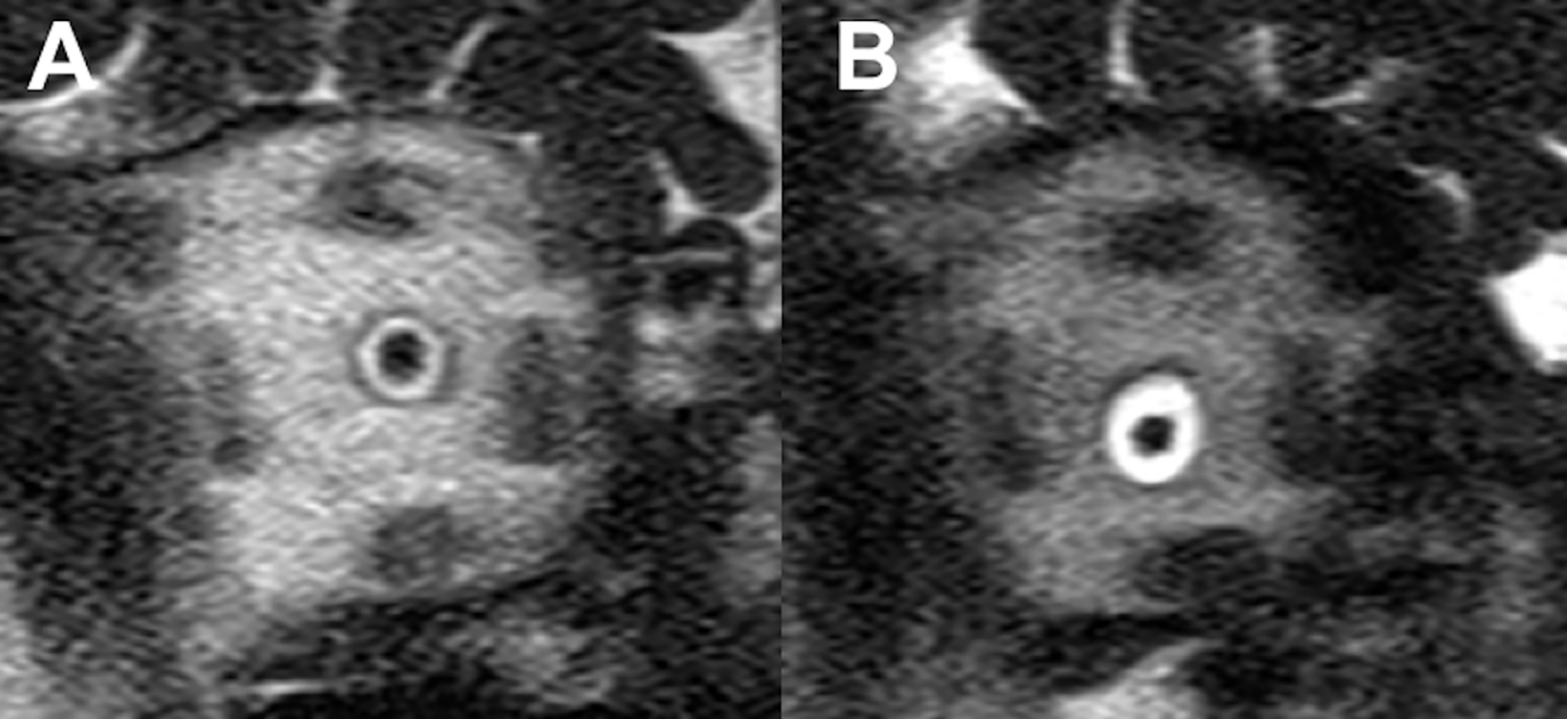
T2-weighted half-Fourier acquisition in single shot turbo spin-echo (HASTE) magnetic resonance imaging scans showing the cross-sectional area of the right intraorbital optic nerve (ION) 5 mm behind the eye. The cerebrospinal fluid can be identified as the hyperintense (bright) ring-shaped area in the center of the scan, and the ION parenchyma as the hypointense (dark) area within the hyperintense ring-shaped area. *A*, ION from a healthy individual; *B*, ION from a glaucoma patient.
